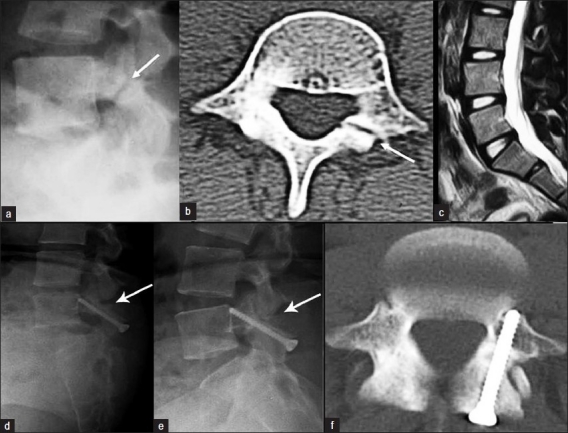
(a) Preoperative lateral radiograph and (b) axial CT scan showing unilateral defect of the pars interarticularis of the L4 vertebra. (c) Sagittal T2 weighted MRI demonstrating the normal L4-L5 disc without any degeneration. Follow-up lateral dynamic radiographs in (d) flexion and (e) extension, showing complete healing of the defect without signs of instability. (f) Postoperative axial CT scan demonstrating complete healing of the spondylolytic defect
